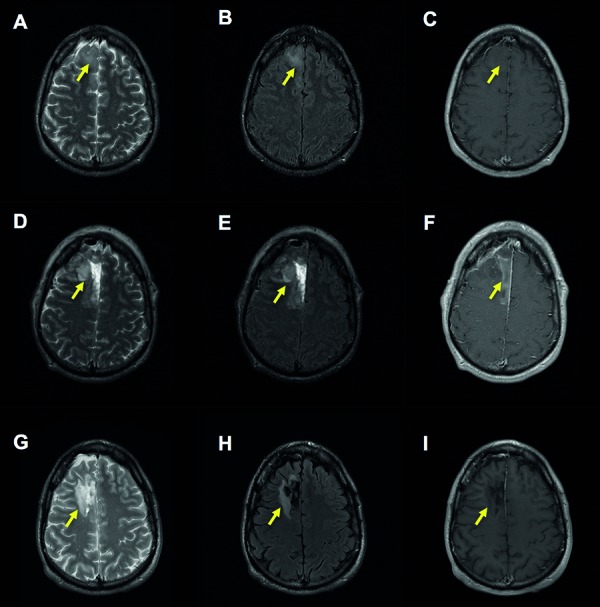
An example of MRI scans in a case with suspected tumor recurrence (case 2). Diagnostic scans (A, B, C) demonstrate a lesion in the right frontal lobe, as indicated by the yellow arrow. Postoperative images (D, E, F) were obtained within 24 hours of surgical resection of a WHO grade II oligodendroglioma (displayed in Figure 2), and display high-signal abnormality around the resection cavity on T2-weighted imaging (D and E) and hemorrhage within the cavity as a T1-weighted hyperintensity (F). Scans suggesting radiological evidence of tumor recurrence are shown (G, H), obtained 23 months from initial resection, the increase in the margin of T2-weighted abnormality surrounding the resection site, as seen in G and H, raised the suspicion of tumor recurrence. No evidence of gadolinium enhancement is seen at this time point (I). A, D, G: T2-weighted image. B, E, H: fluid attenuated inversion recovery (FLAIR) image. C, F, I: T1-weighted image post administration of gadolinium.
